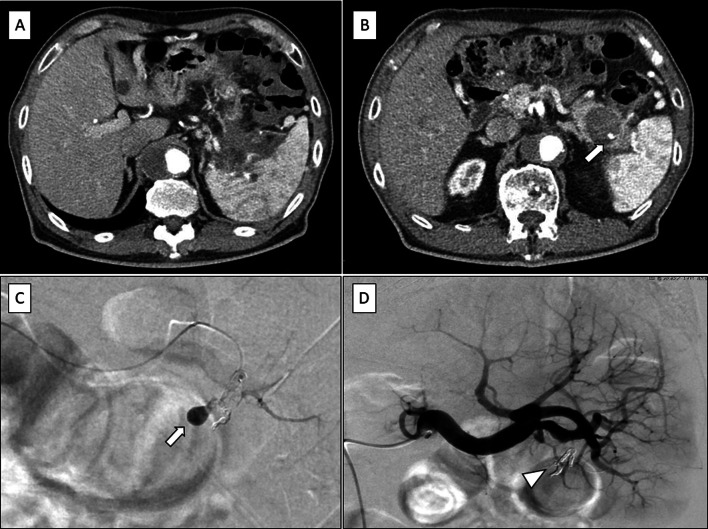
A Contrast-enhanced computed tomography showing fluid collection around the pancreatic tail. B With pseudoaneurysm of the splenic artery (arrow). C Abdominal angiography showing pseudoaneurysm of the splenic artery (arrow). D Transcatheter arterial embolization was performed (arrowhead)
